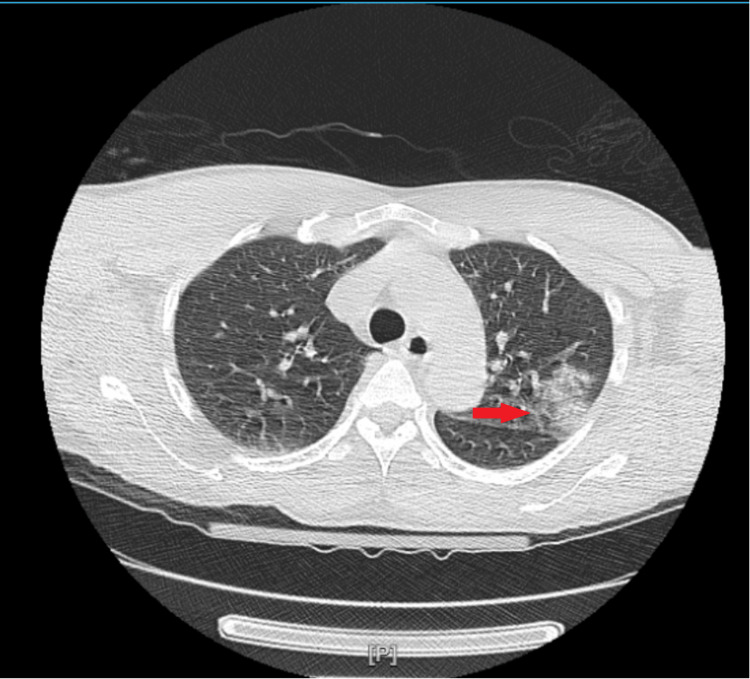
CT scan of the chest showing the red arrow pointing at the left upper lobe consolidation
